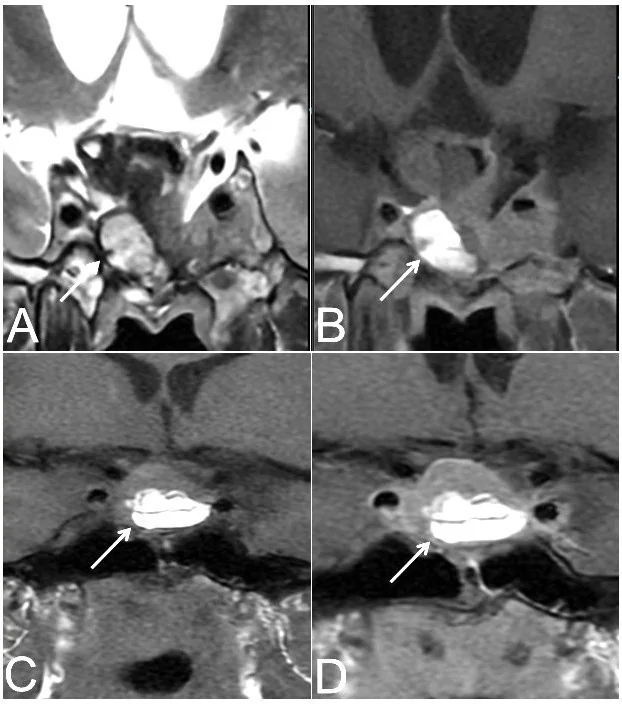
Expected Post-surgical MRI Findings. Surgical packing materials after transsphenoidal surgery. 3 months post transsphenoidal surgery MRI is shown on coronal T2WI (A) and unenhanced coronal T1WI (B). The images demonstrate a typical postoperative fat flap (arrow) along the right side of the sella turcica, corresponding to a serum IGF-1 level of 15 ng/mL (within normal range) consistent with expected postoperative imaging observations. Another patient with acromegaly, status post transsphenoidal surgery, is shown on unenhanced coronal T1WI (C) and contrast-enhanced coronal T1WI (D). The images demonstrate a similar gelfoam/fat flap (arrow) within the sella turcica.
